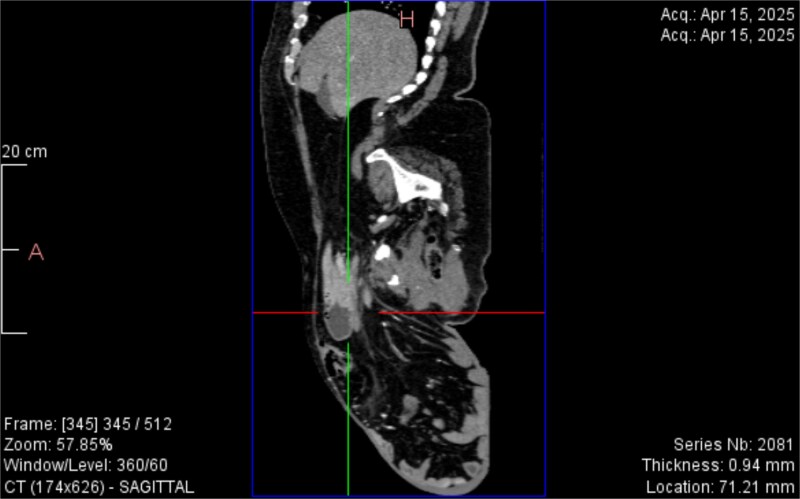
Sagittal CT view inguinal hernial sac with pancreatic head and D2 and D3 segment of duodenum.
